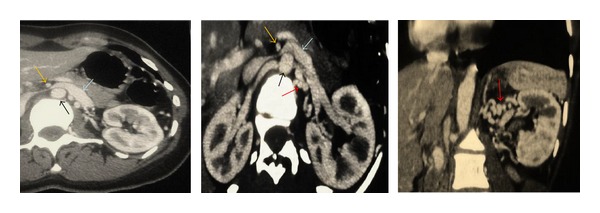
CTA of the abdomen and pelvis showed complete disappearance of the renal vein thrombosis and demonstrated the nutcracker syndrome: compression of the LRV between the aorta (black arrow) and the SMA (yellow arrow), with a dilatation of the hilar portion of the LRV (blue arrow). The red arrow showed and surrounding vascular collaterals.
